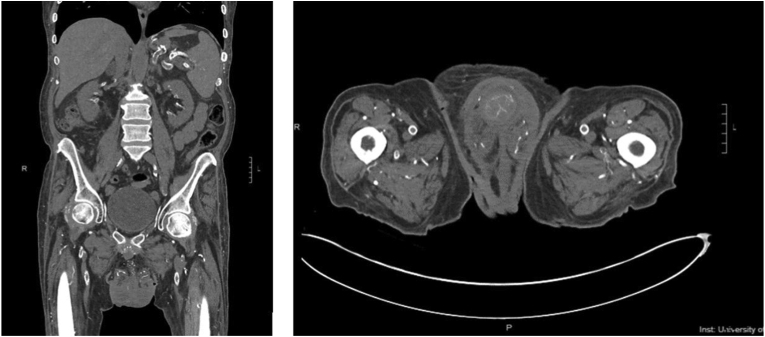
Non-Contrast computed tomography scan of patient's abdomen and pelvis: Significant microvascular calcification within large and small order vessels of the patient's genitals and pelvis, including extensive calcifications of penile vasculature.
